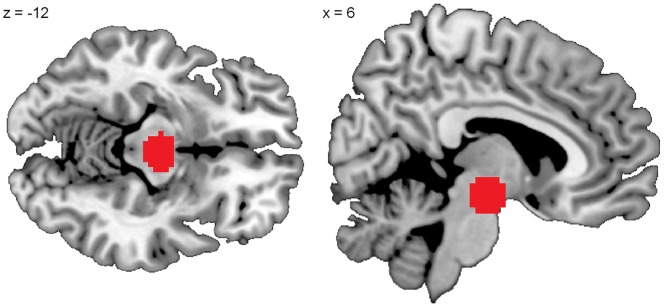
Mask for the ventral tegmental area (VTA): The region of interest was drawn manually on MRI-based anatomy using an anatomical atlas (Duvernoy HM (1995)).
